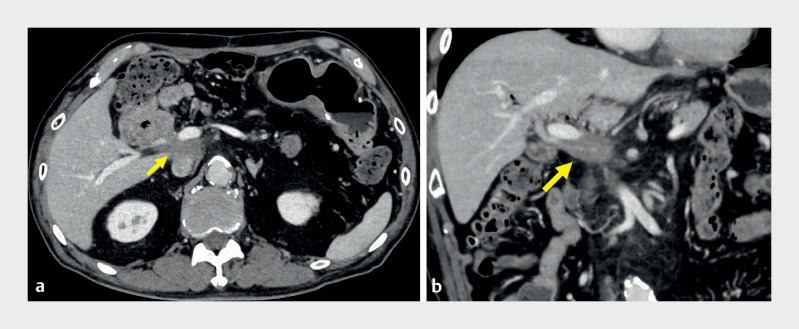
Contrast-enhanced computed tomography showing a 30-mm hypovascular mass (arrow) behind the portal vein.
